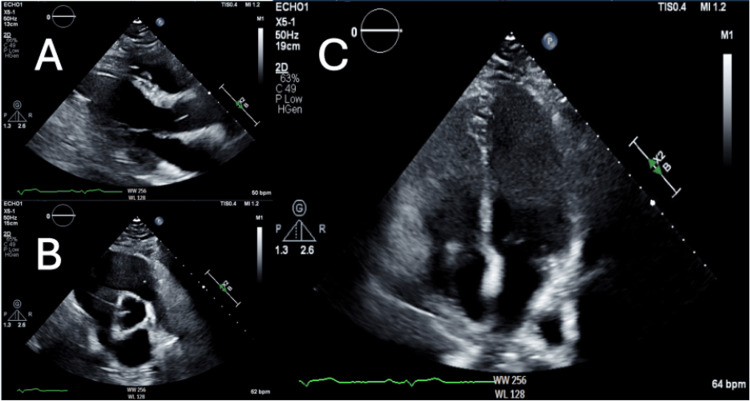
Transthoracic echocardiogram (A) Transthoracic echocardiogram, long axis, demonstrating normal wall thickness and aortic root. (B) Basal short axis view demonstrating normal aortic root. (C) Apical four-chamber view demonstrating normal chamber sizes
